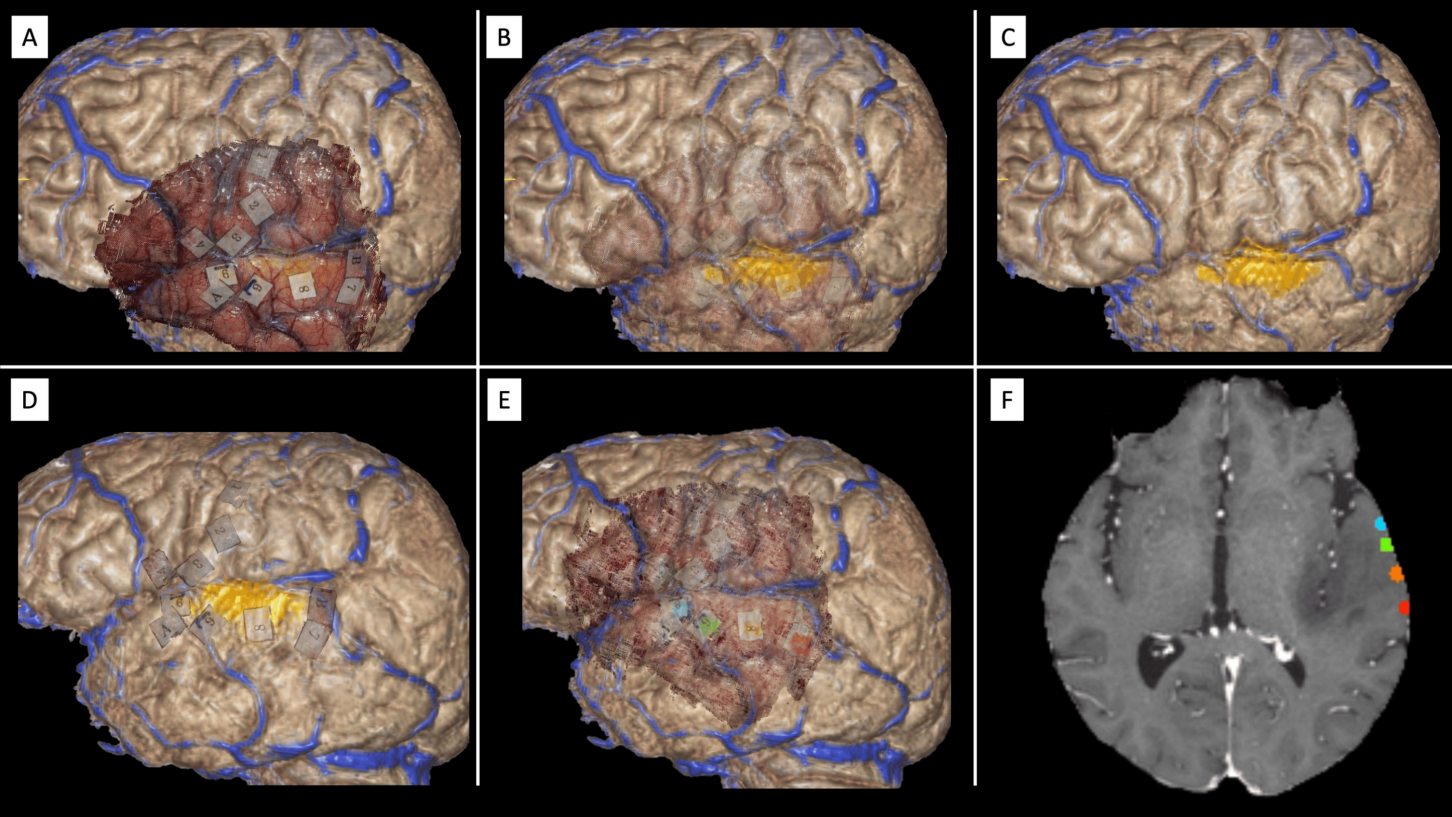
Demonstrative clinical application 1. (A) In the same patient from Figure 4, the Multimodal Optico-Radiological Image (MORI) integrates the surgical field, including cortical map tags, with a 3D-rendered brain extracted from pre-operative MRI. (B and C) Adjusting the opacity of the optical images allows visualization and verification of the alignment accuracy between the optical and radiological modalities. In this case, the venous system alignment between both modalities is confirmed to be accurate. (D and E) Using visualization software (NewVes), selected cortical tags can be color-coded, extracted, and exported for further analysis. (F) The extracted tags are superimposed onto a 2D radiological image for enhanced visualization. In this example, tags 6-9 in the superior temporal gyrus are color-coded as green, red, orange, and blue, respectively. For a better visualization experience watch Video 2. The image is copyrighted by the author (Masazumi Fujii) of this study (licensed under CC BY-ND 4.0).
